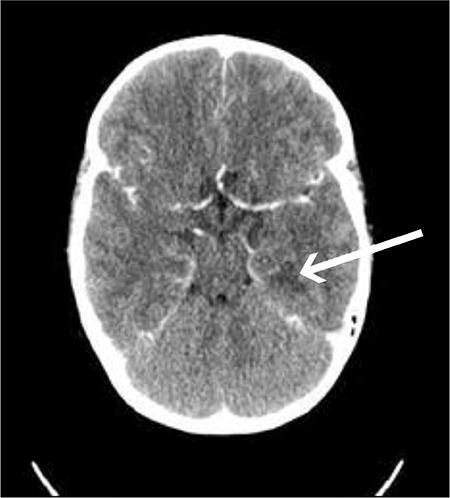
Brain computed tomography scan. Thirteen mm hypodense lesion in the left temporal lobe, not visible after contrast injection-ischemic lesion? The supratentorial ventricular system is narrow and symmetrical. Cerebral sulci are not distinct
